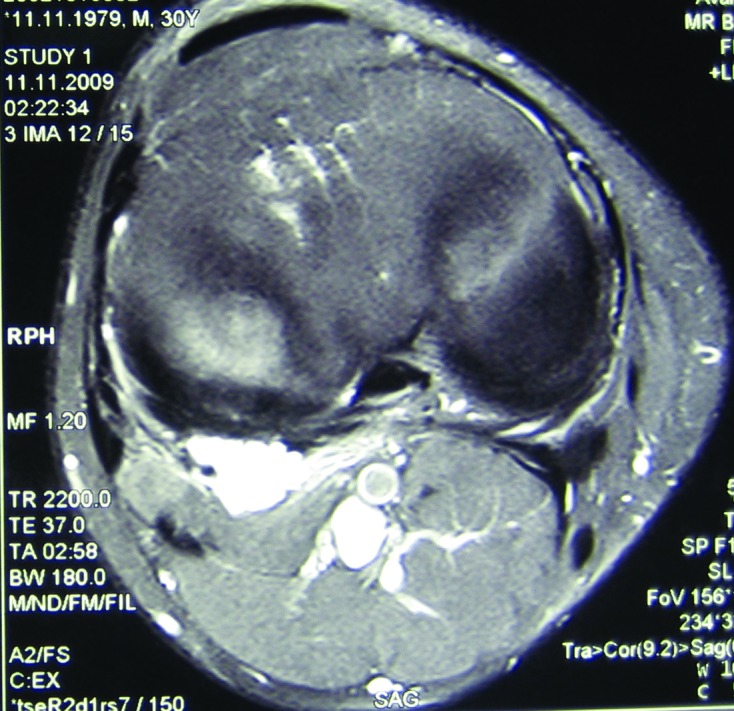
Perimeniscal cyst is seen on the axial MR imaging of the posterior horn of the lateral meniscus of right knee.
